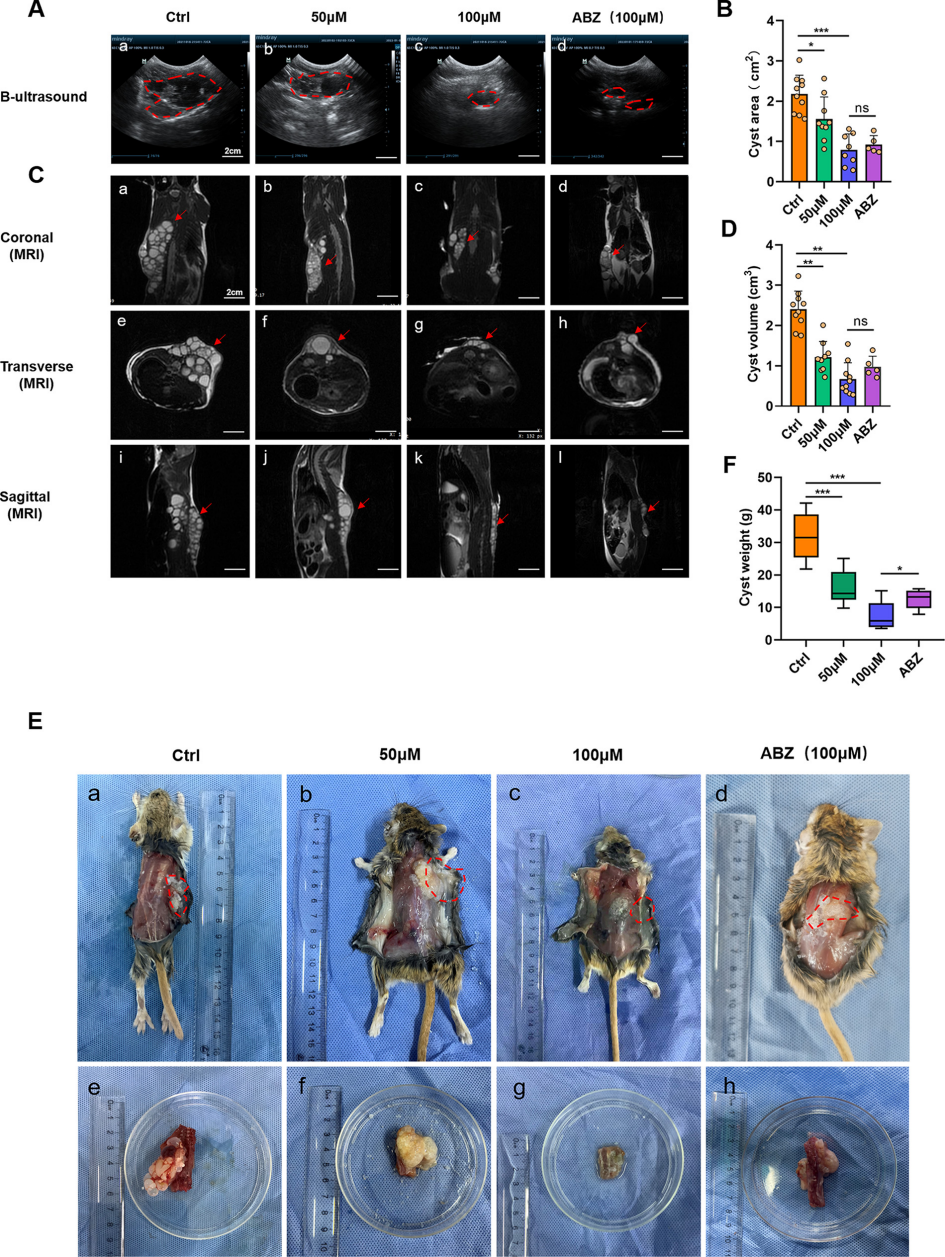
α-MG is effective against spinal CE in gerbils. (A) The gerbils were infected with 3,000 *E. granulosus* PSCs and intraperitoneal injection with 50 µM, 100 µM α-MG in different groups for 8 weeks (twice a week). A total of 100 µM ABZ was used as a positive control. Equal amount of solvent injection was used as control. The location, shape, boundary, and internal echo of abnormal echo focus, blood flow signal, as well as maximum lesion diameter were recorded by B-ultrasound. Clear image was reserved to calculate the lesion area (cm^2^). (B) The cross-sectional area of the cysts in the ultrasound images were calculated and compared. (*n* ≥ 5). (C) Images of sagittal, coronal plane, and transverse section were captured by MRI. Red arrow indicates CE tissue. (D) The volumes of cysts in MRI pictures were calculated and compared. (*n* ≥ 5). (E) Spinal hydatid tissue was isolated and the cysts were weighed after thorough dissection. (F) Weight of cysts in different groups were measured. (*n* ≥ 5). Results shown are represented as mean ± SD. *, *P* < 0.05; , *P* < 0.01; *, *P* < 0.001 using the two-sided Student’s-*t* test.
